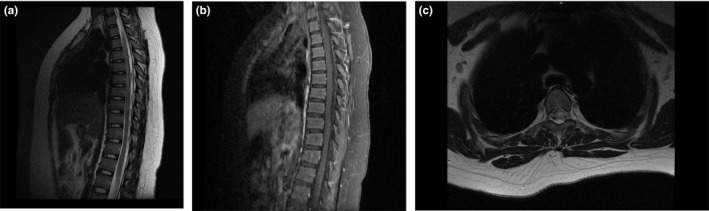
MRI‐scan 4 days after symptom onset. Thoracic cord MRI image showing areas of T2 prolongation in the central spinal cord. (a) Sagittal T2. (b) Sagittal T1 Gd‐enhanced. (c) Axial T2
